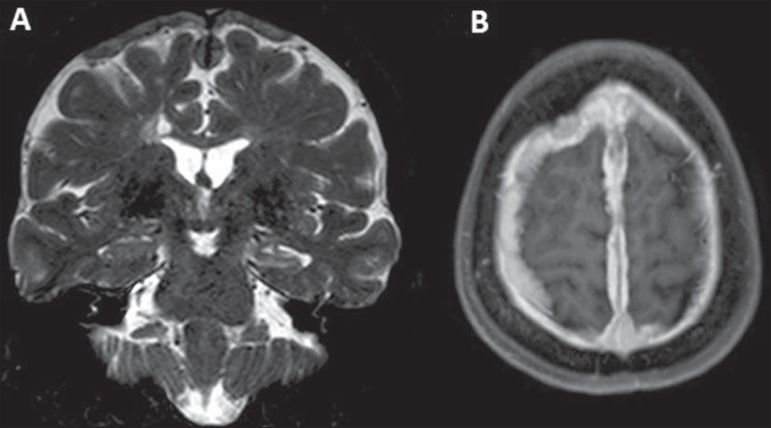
A: Coronal magnetic resonance imaging – T2-weighted sequence
